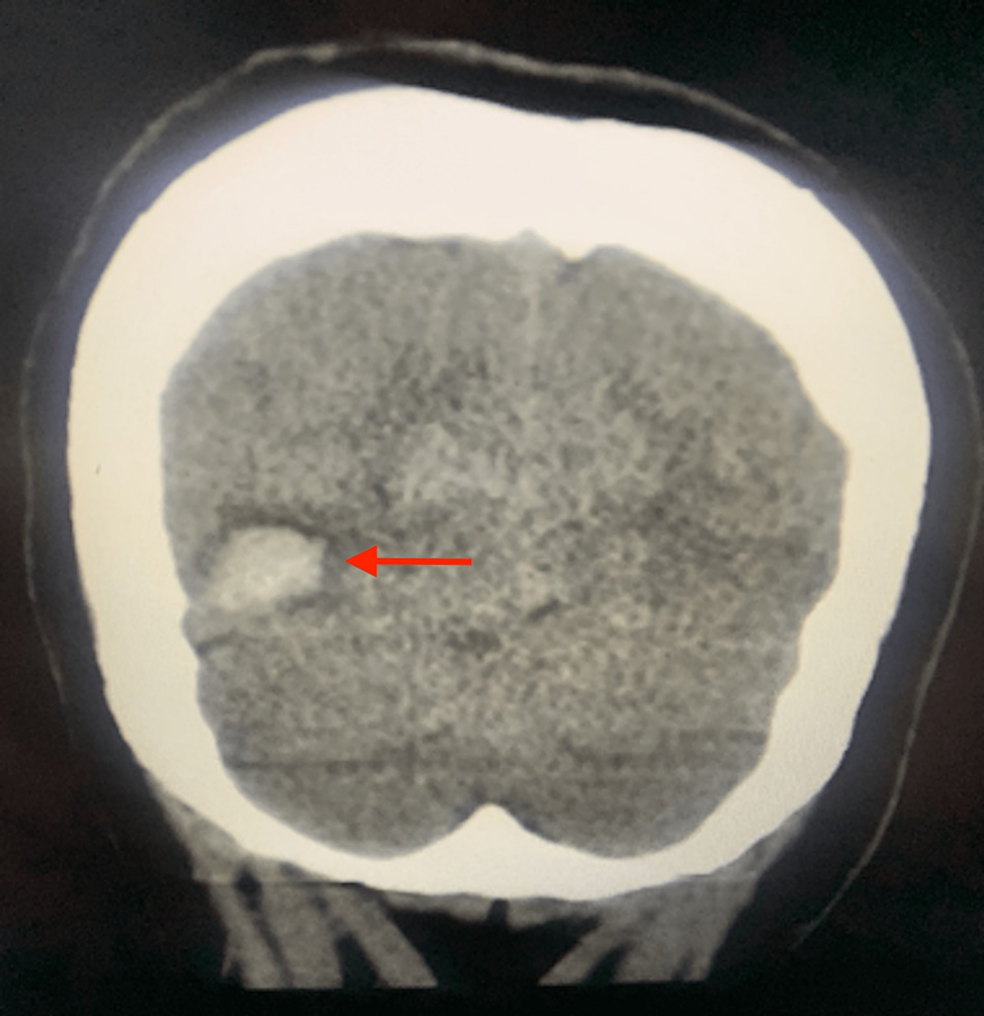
Coronal section from the initial brain CT without contrast done in the emergency department showing a right occipital hematoma
